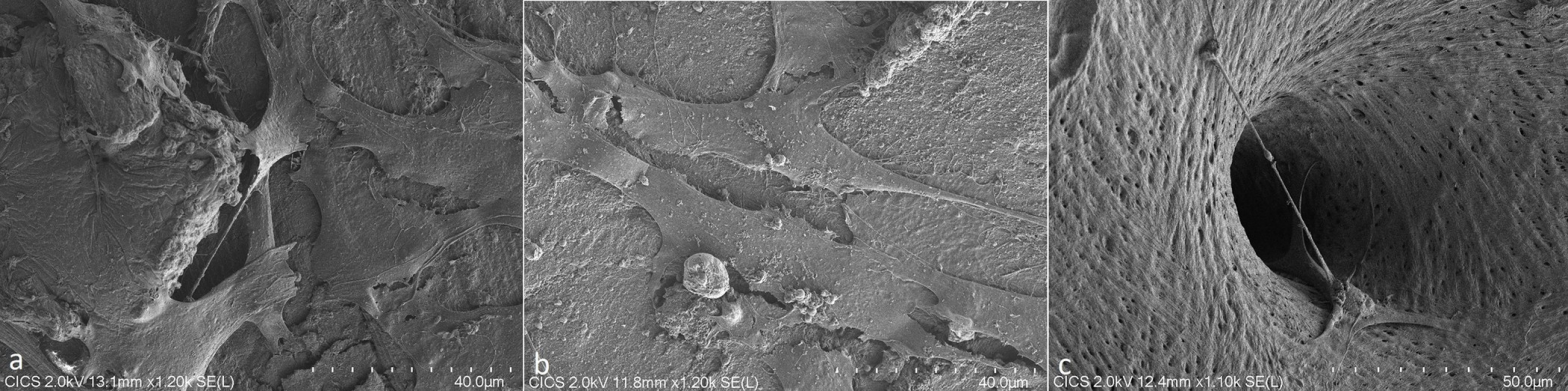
SEM images (x1200 magnification) of osteoblasts on the 3 different bones after 4 days of culture (a: « F-bone », b: « scCO2-bone », c: « CT-bone »).
